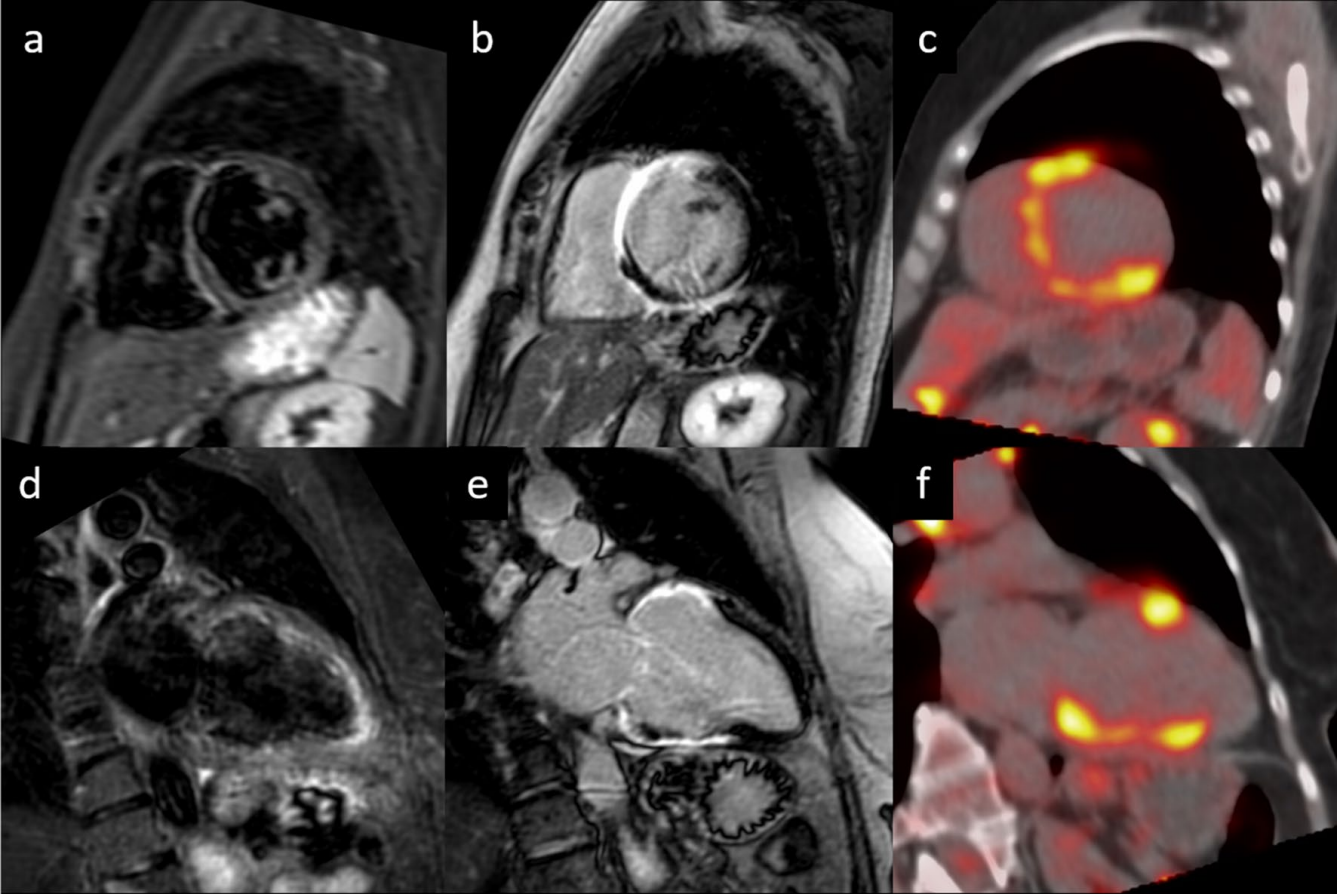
57-year-old female with frequent syncopal episodes and ventricular tachycardia, LV dilation and severe reduction in EF at TTE, with no obstruction of coronary arteries at coronary angiography. CMR revealed no edema on T2w-STIR images (a short axis view and d LV long axis view) and extensive areas of late gadolinium enhancement at IR-TFE images (b short axis view and e LV long axis view), with a non-ischemic pattern of distribution. The FDG-PET (c short axis view, f long axis view) confirmed the diagnosis of sarcoidosis with the identification of areas of FDG uptake (i.e., active inflammation) within the myocardium and in the mediastinal lymph nodes. *LV* left ventricle, *EF* ejection fraction; *TTE* transthoracic echocardium
